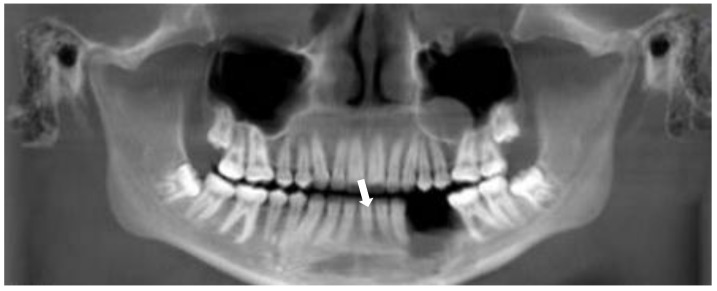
Pre-operative radiograph demonstrating ill-defined radiolucent lesion in left anterior-to-premolar mandibular body. White arrow indicates lesion.
